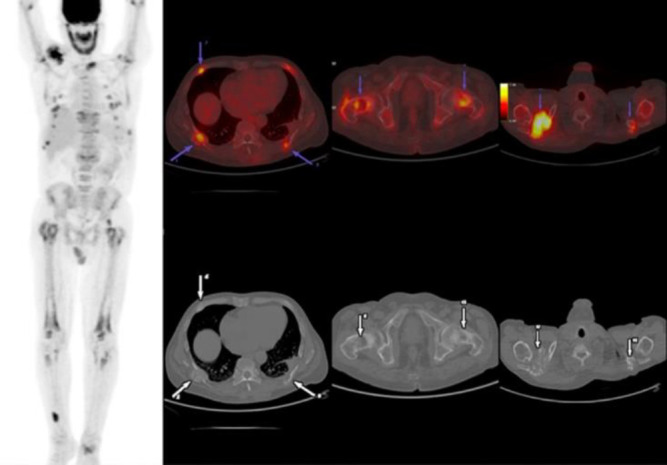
Whole-body anterior maximum intensity projection (MIP) images from [^18^F]-FDG PET/CT demonstrated diffusely increased uptake in the axial and appendicular skeleton, with absent of radiotracer excretion in the kidneys and urinary bladder. Axial CT and fused PET/CT images showed multifocal lytic lesions with increased uptake in clavicles, right scapula, bilateral ribs, multiple vertebrae, pelvic bones and right tibia indicating multifocal osteitis fibrosa cystica (SUV_max _up to 9.9, measuring up to 8×5×7cm in right scapula)
